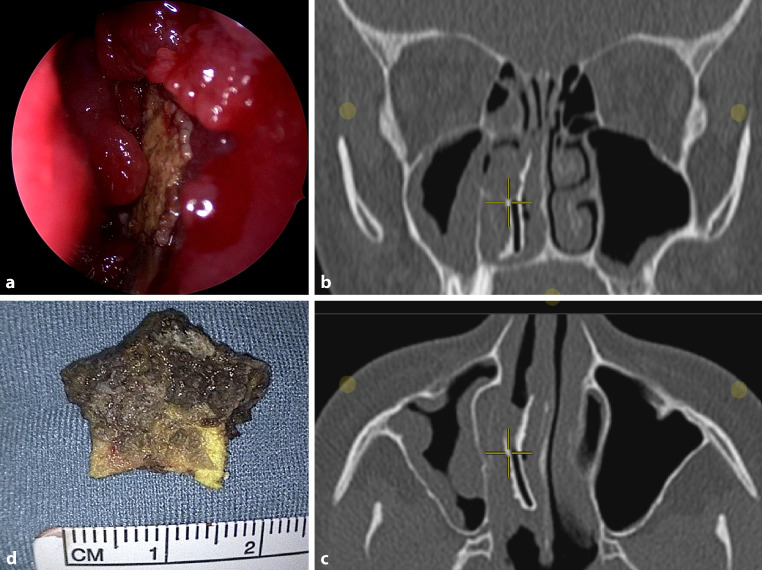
a Anterior rhinoscopy. b, c Computed tomography imaging of the paranasal sinuses (b coronal plane, c axial plane). The crosswire indicates a vertically situated hyperdense foreign body with surrounding soft tissue density in the right common middle meatus. d Removed foam star from the nasal cavity
